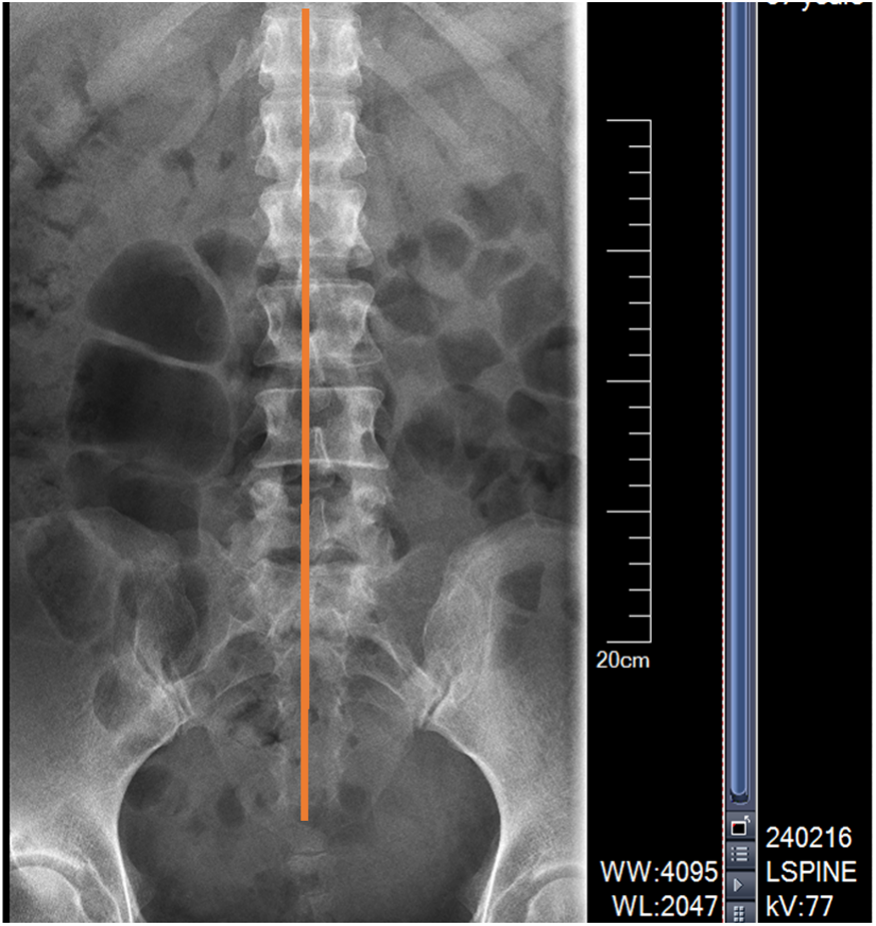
X-ray anteroposterior view indicates: spinous process is not centered, showing signs of rotation.
